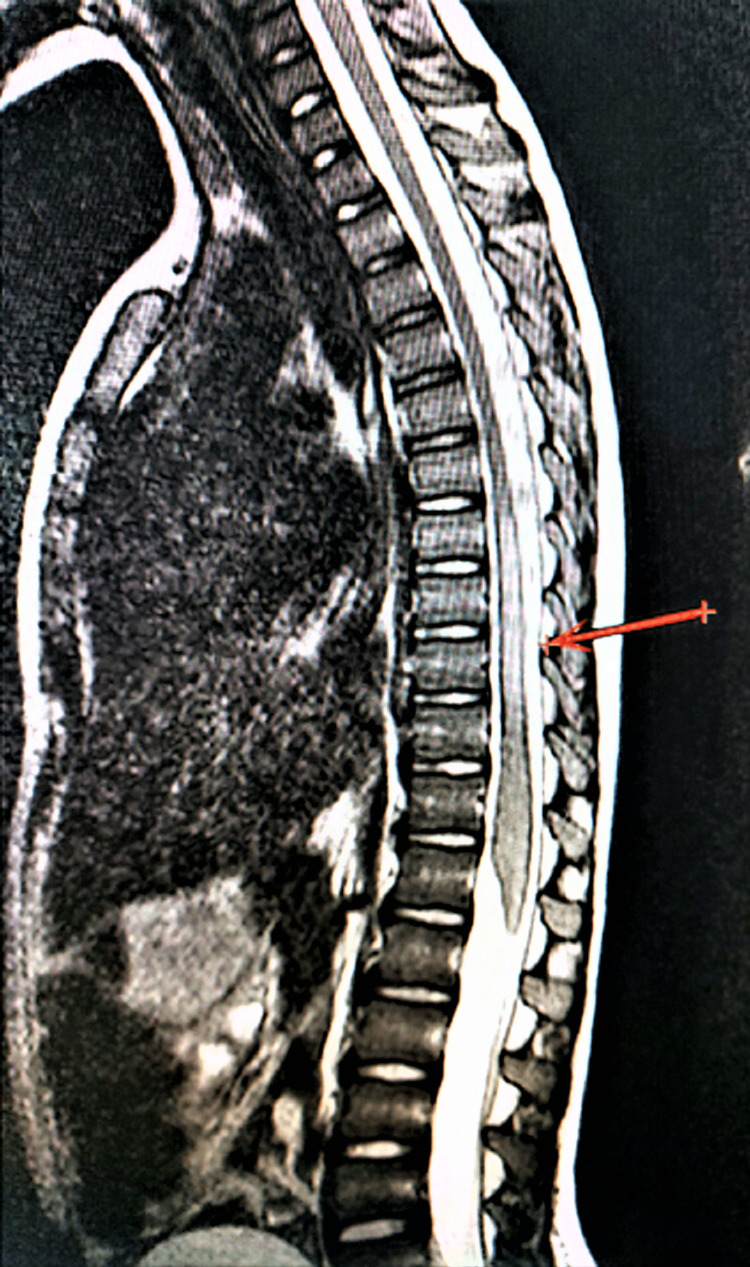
MRI of the spinal cord of a patient at the time of diagnosis of schistosomal myeloradiculopathy T1-weighted sequences after intravenous contrast administration show intense granular and heterogeneous impregnation of the low thoracic spinal cord, conus medullaris, and cauda equina roots in sagittal projections (red arrow).
